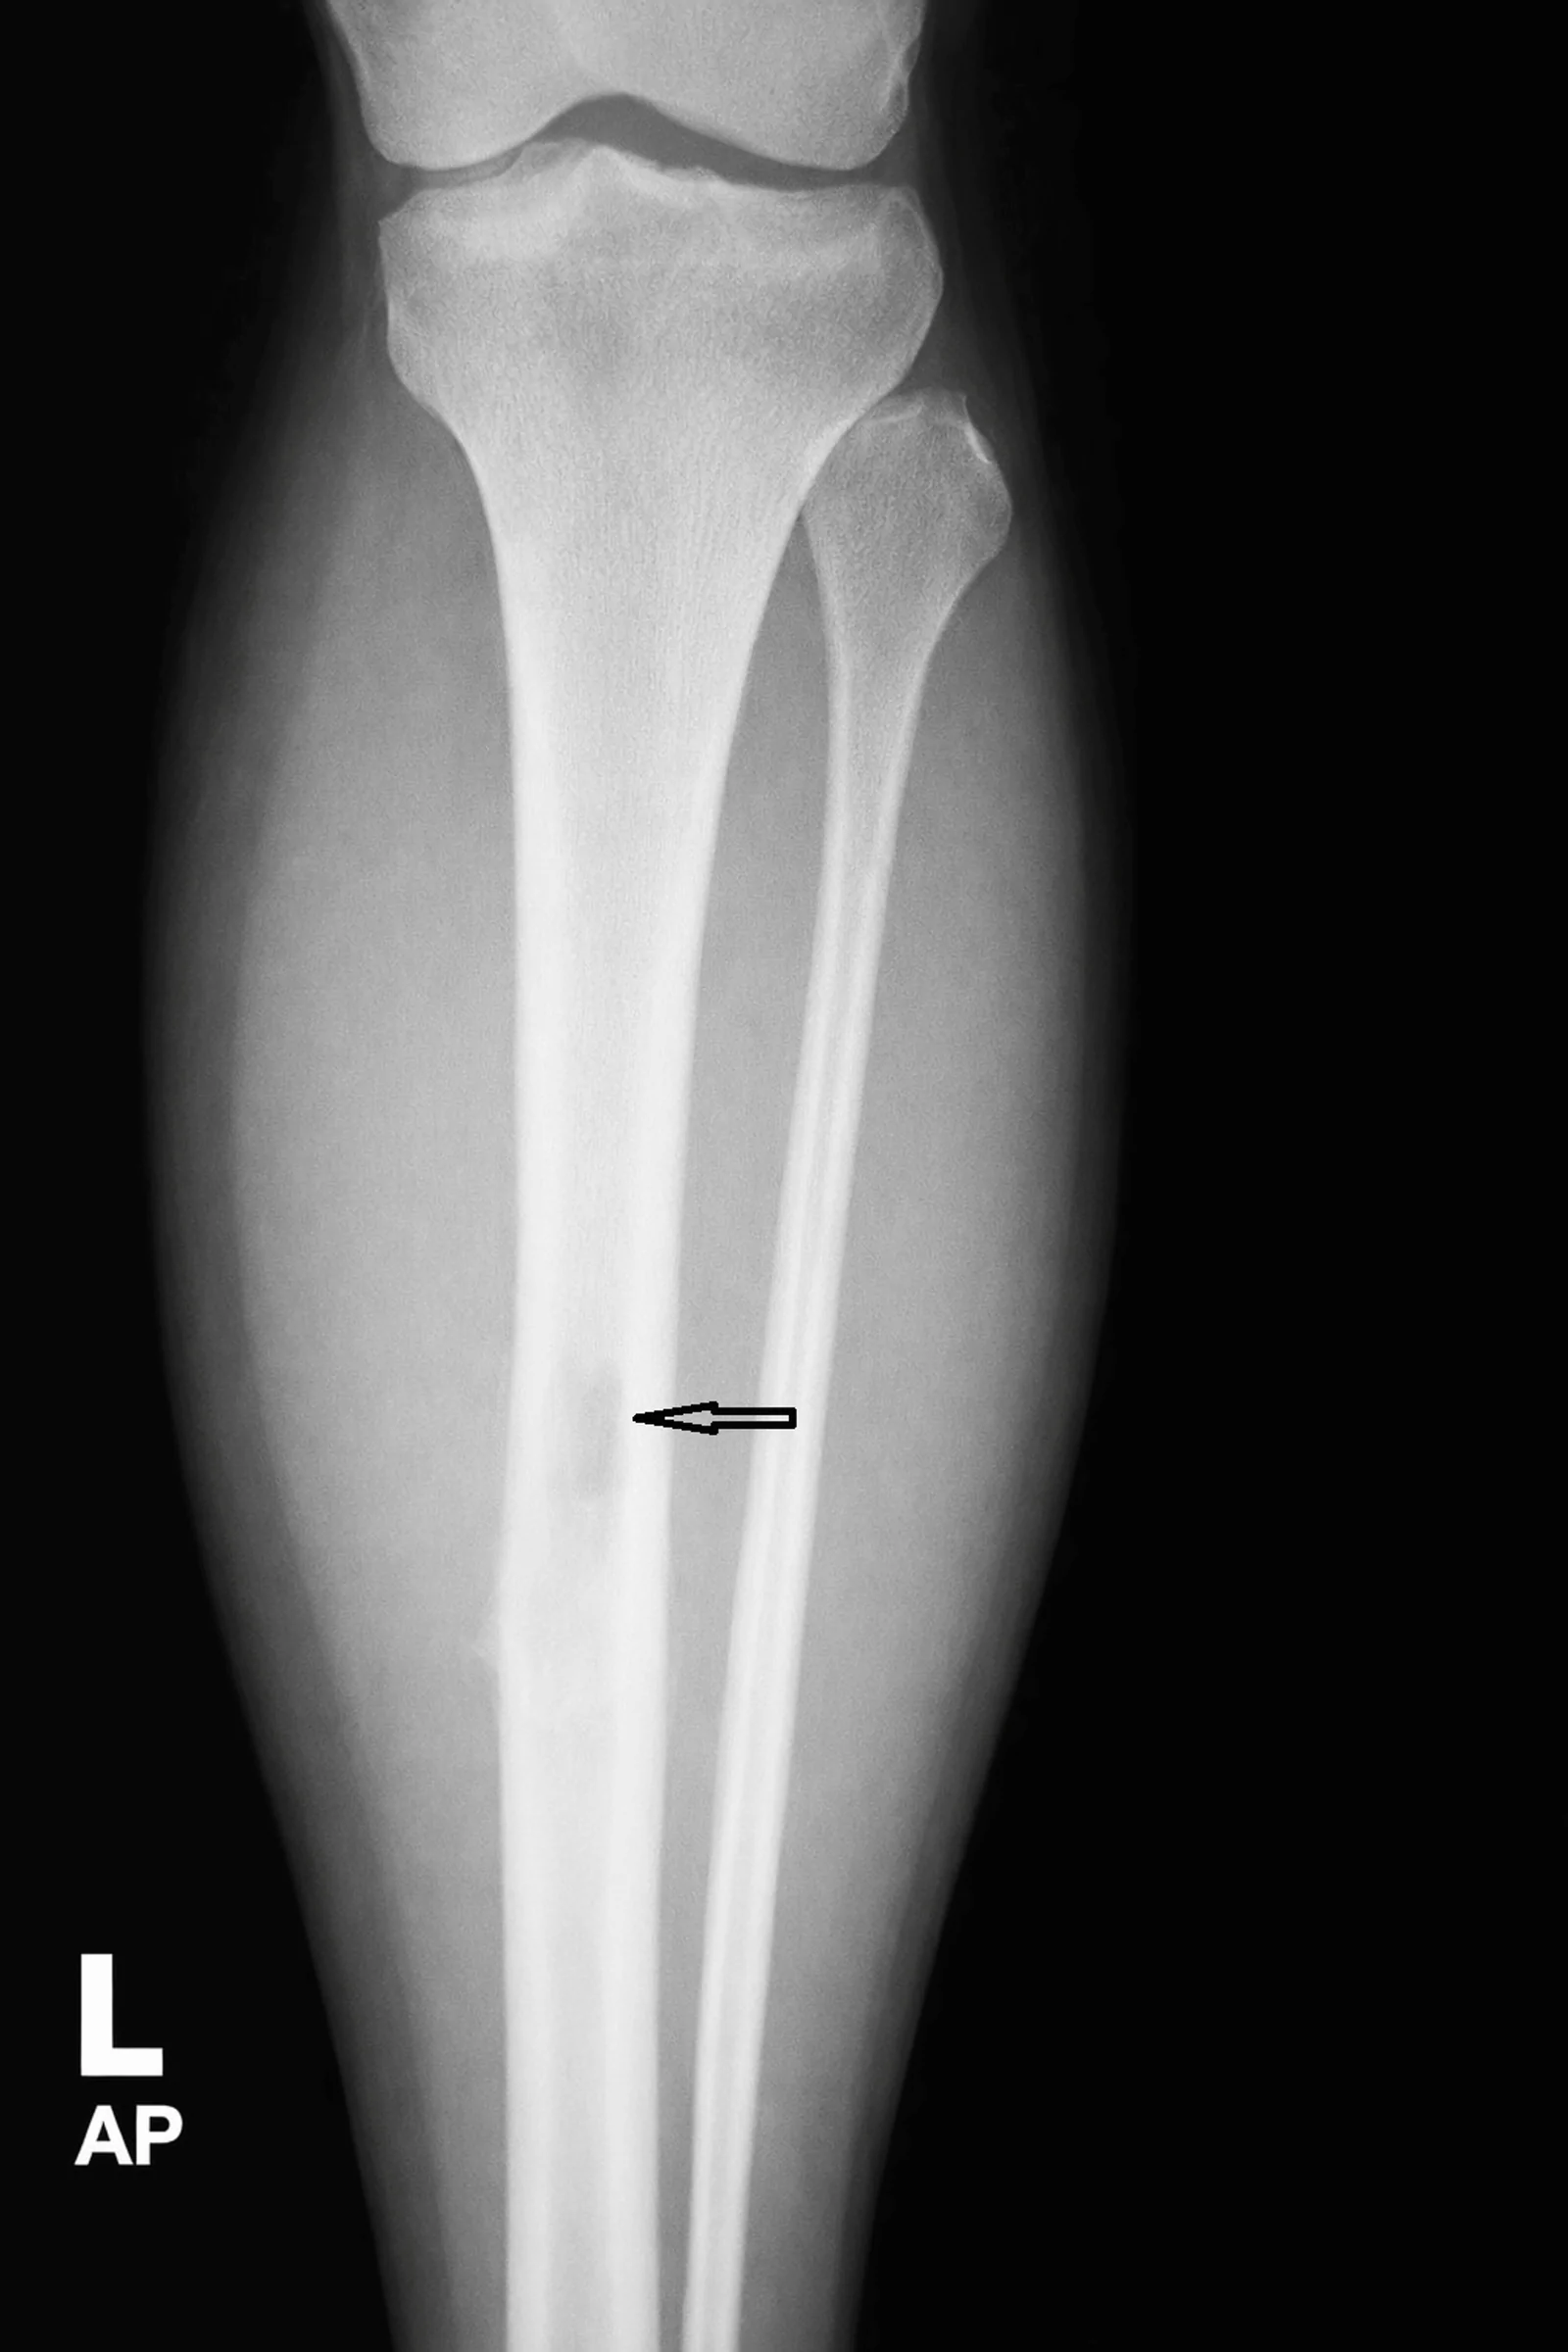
Anteroposterior (AP) radiograph of the left tibia demonstrating adamantinoma. The radiograph displays the AP aspect of the adult left tibia and fibula. The black arrow points to a well-defined, eccentric, expansile lytic lesion located in the diaphysis of the tibial shaft. The lesion has a slightly ground-glass appearance and is surrounded by a thin, sclerotic rim, consistent with imaging characteristics of an adamantinoma. Soft tissue structures and the adjacent fibula are visible. Note the specific medullary location. Source: Original image owned by the authors.
